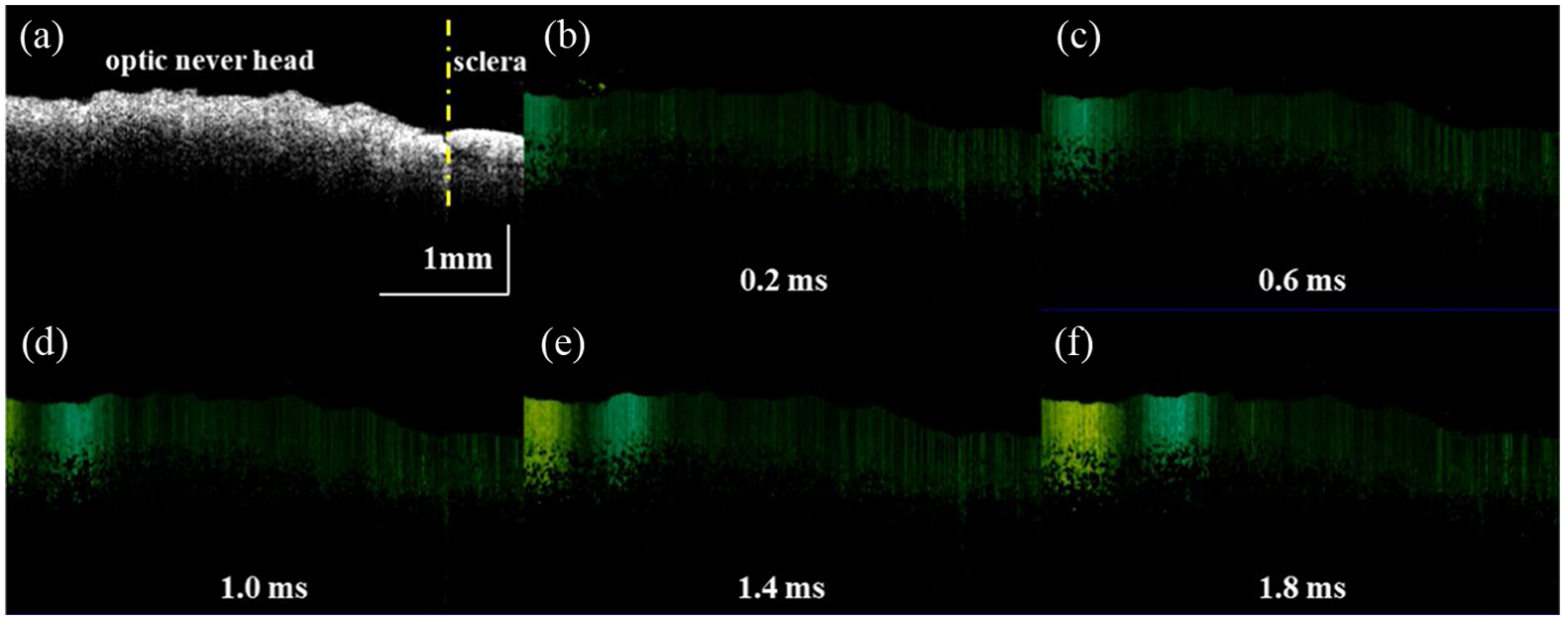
The elastography of the *ex vivo* ONH. (a) The B-scan OCT image of the ONH, (b)–(f) Doppler OCT B-scan images of the ONH at 0.2, 0.6, 1.0, 1.4, and 1.8 ms.
